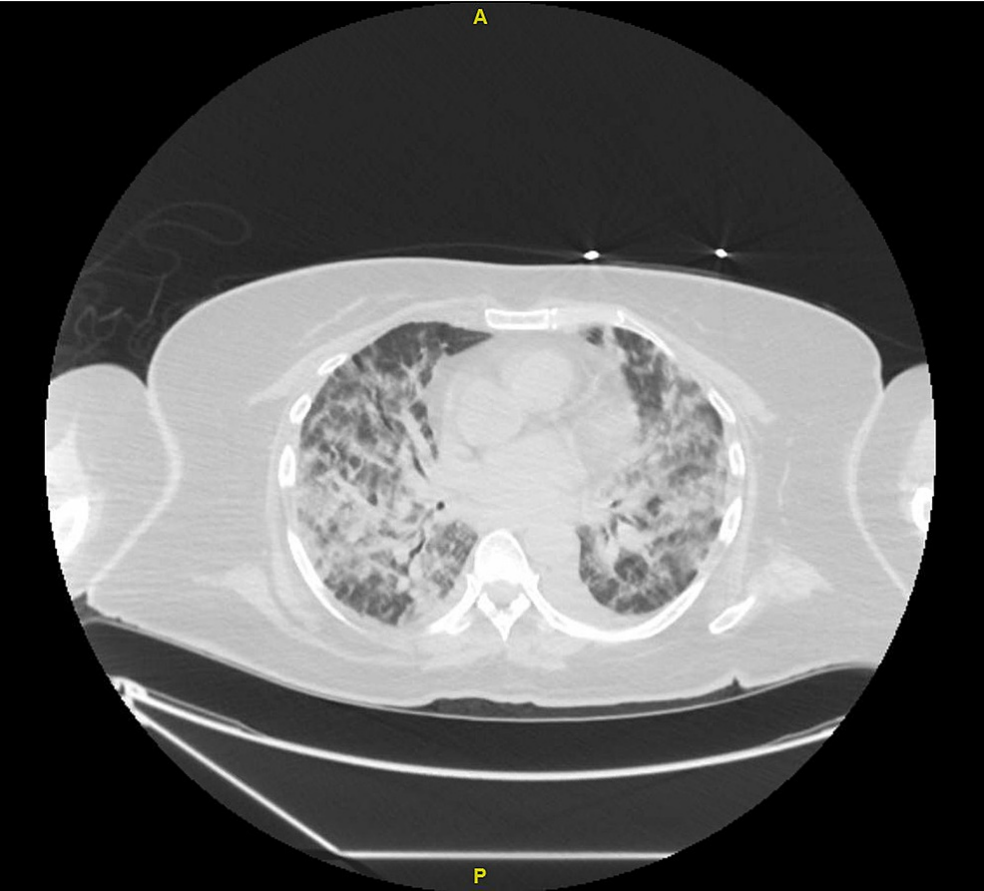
Computed tomography (CT) of chest showing bilateral diffuse patchy infiltrates compatible with COVID-19 pneumonia
